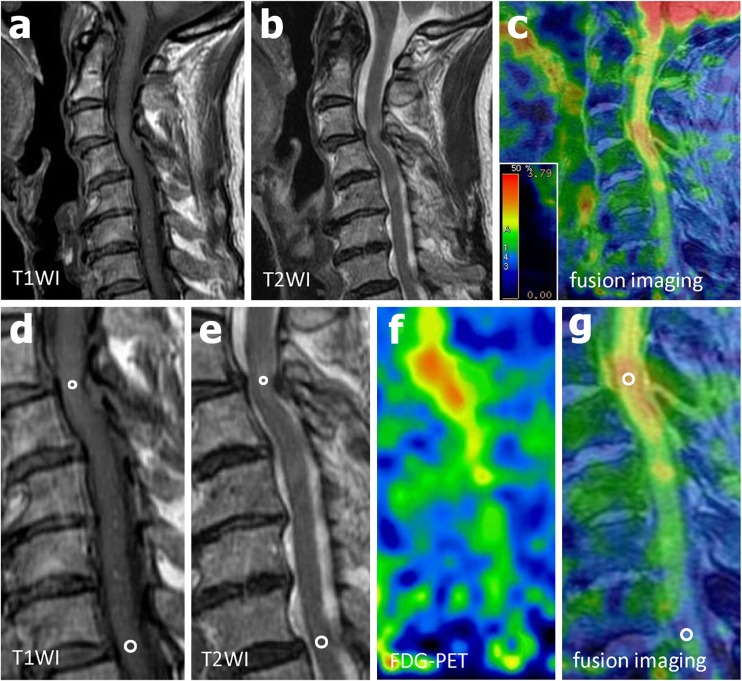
Patient 22. An 80-year-old woman with cervical spondylotic myelopathy was treated surgically and showed a neurological improvement rate of 75.0 % at follow-up. a Midsagittal T1-W MR image. b Midsagittal T2-W MR image. c MRI/PET fusion image. d T1-W MR image for calculation of SIR as the ratio of the signal intensity in the midsagittal area of increased signal intensity (*small circle*) to the signal intensity in the midsagittal area of normal signal intensity at the level of the C7/T1 disc (*larger circle*), e T2-W image for calculation of SIR in a similar manner. f Sagittal ^18^F-FDG PET image. g Sagittal MRI/PET fusion image demonstrates a focal increase in ^18^F-FDG uptake at the level of a lesion showing increased signal intensity. SUVR is calculated as the ratio between the SUV_max_ of the lesion (*circle* diameter 4 mm) and the SUVmean of the normal cord at the level of the C7/T1 disc (*circle* diameter 4 mm)
